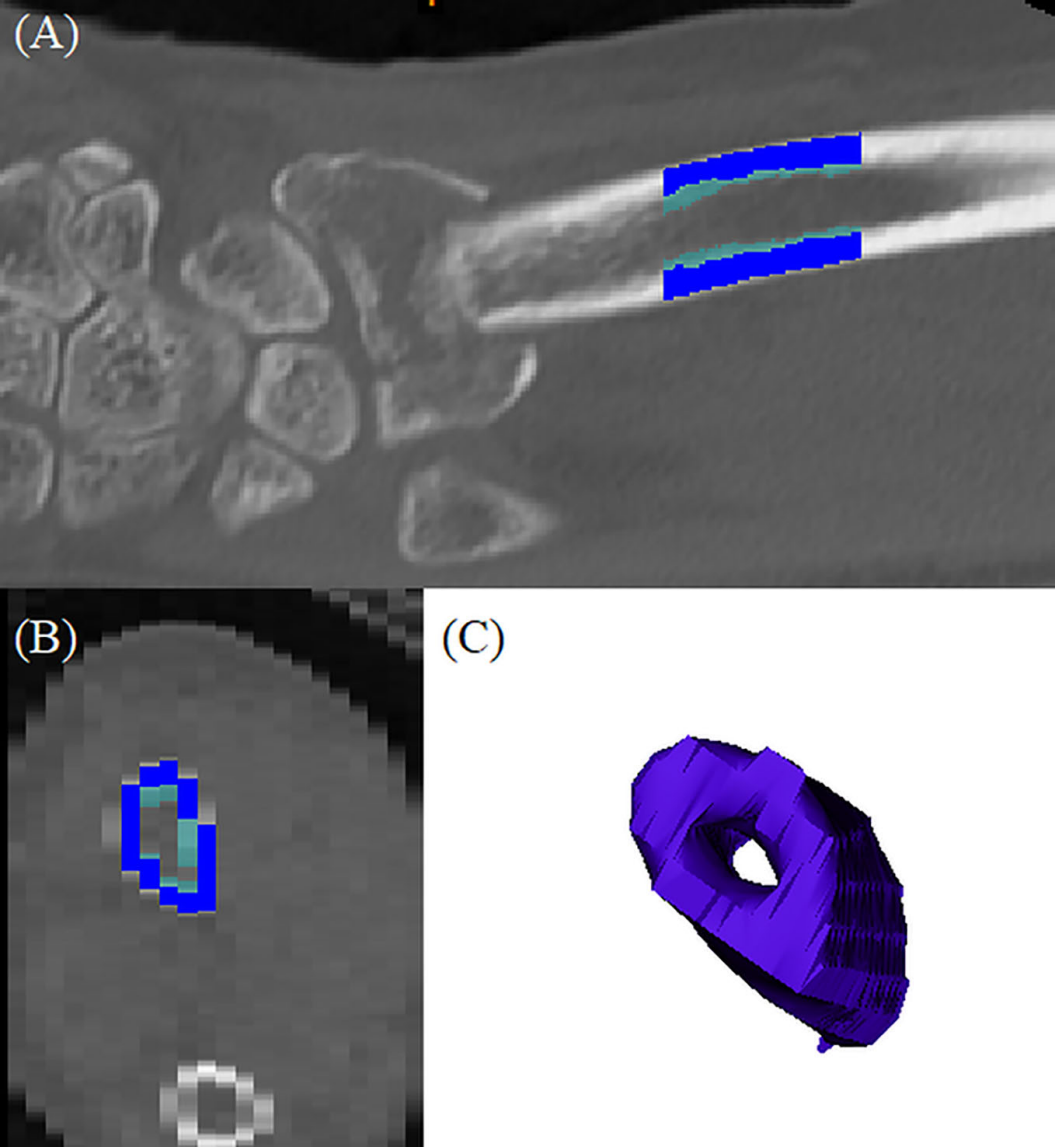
3D reconstructed distal radius bone model: (A) coronal section of the original CT images; dark blue indicated cortical bone and light blue indicated trabecular bone, (B) axial section of the original CT image; dark blue indicated cortical bone and light blue indicated trabecular bone, (C) reconstructed bone model.
